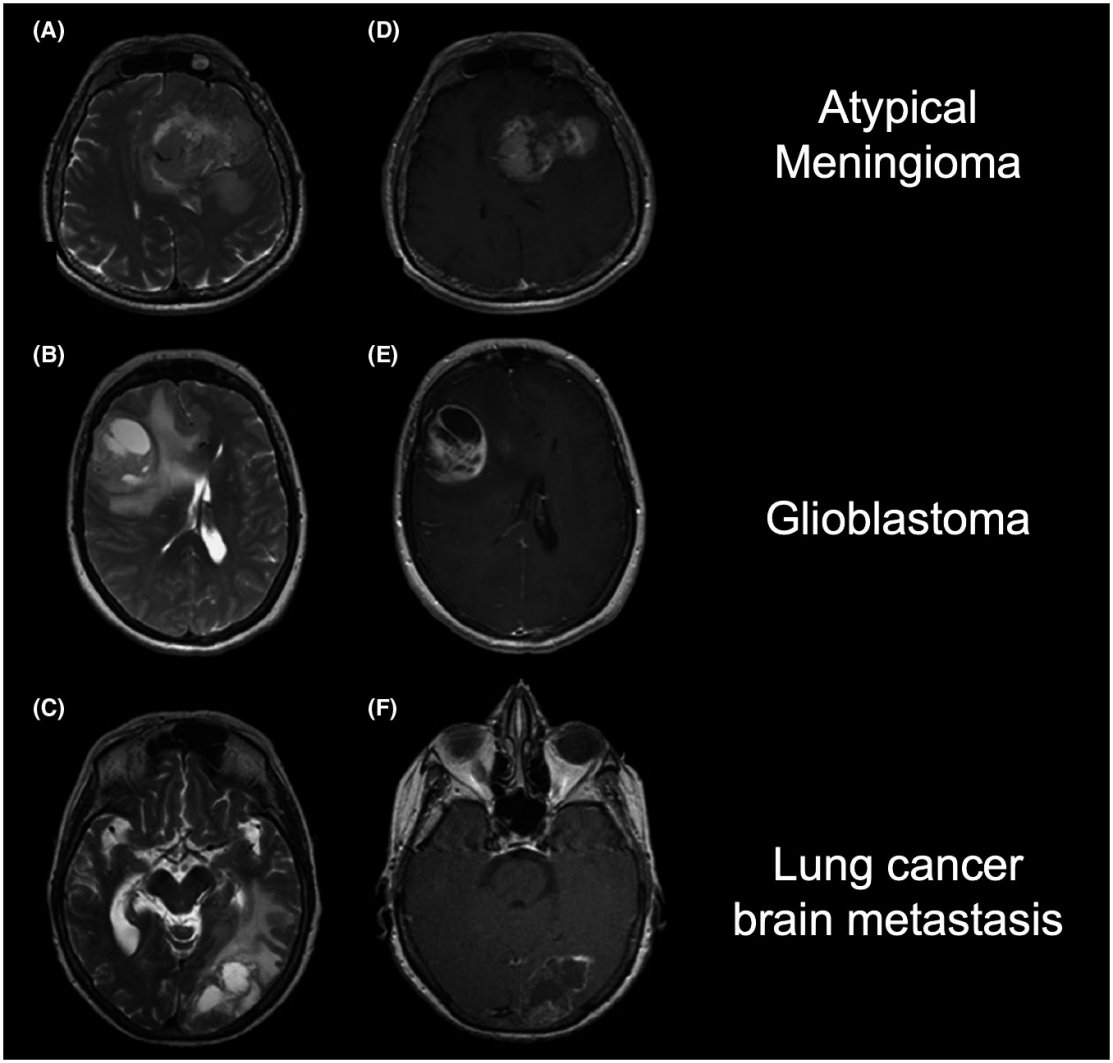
Representative T2‐weighted (A–C) and contrast‐enhanced T1‐weighted MR images (D‐F) of an atypical meningioma (top row), a glioblastoma (middle row), and a lung cancer brain metastasis (bottom row). Notably, the similar radiological findings make it difficult to differentiate between these three tumor entities. Here, advanced neuroimaging techniques may provide additional diagnostic information to improve differential diagnosis. Adapted from Svolos et al. [101], with permission from Elsevier
